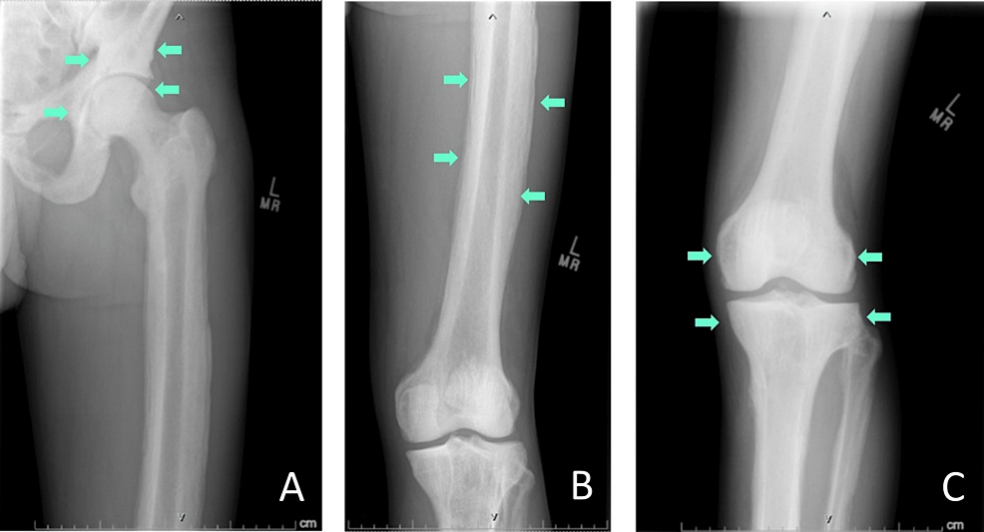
X-rays of the left hip (A), left femur (B), and left knee (C) showed diffusely increased sclerosis and cortical expansion of all visualized bones
